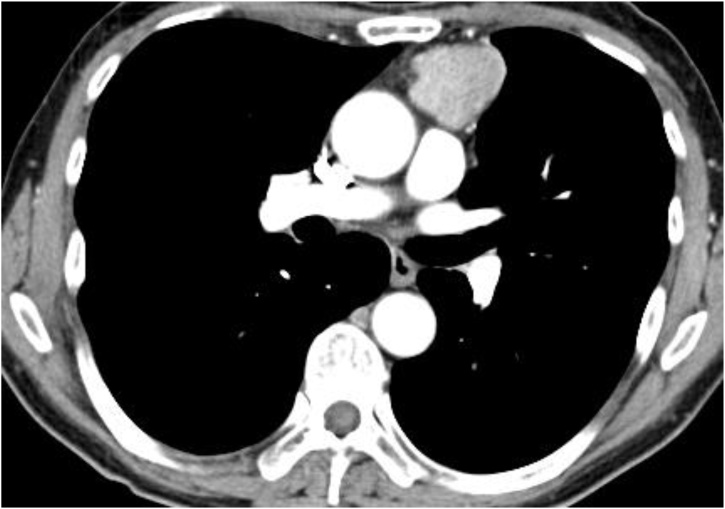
(A, B) Chest computed tomography. A 4.5-cm-diameter thymic tumour.
